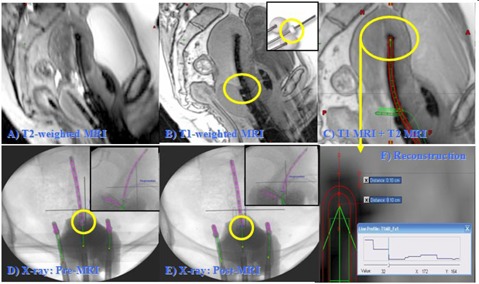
Reconstruction of titanium T&O applicator on MR images: (a) T2‐weighted MRI with 3 mm slice thickness; (b) T1‐weighted MRI with clear flange information; (c) fused MRI (T1‐ and T2‐weighted); (d) X‐ray prior to MR scan; (e) X‐ray following MR scan; (f) tandem tip of reconstructed applicator is described in detail on MR images with MR intensity profile. The flange position in (d) and (e) enables to calculate Δd. From the maximum intensity change point, we account for 1 mm artifacts and another 1 mm for catheter thickness.
